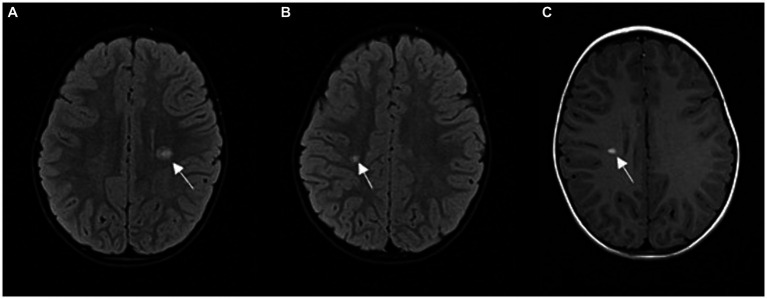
(A,B) FLAIR sequence in axial plane at two different levels; hyperintense demyelinating lesions (A) periventricular, above the left lateral ventricle (arrow), (B) in the deep white matter of the right centrum semiovale (arrow); (C) T1 contrast enhancement sequence in axial plane; homogeneous contrast enhancement of an active lesion (arrow).
